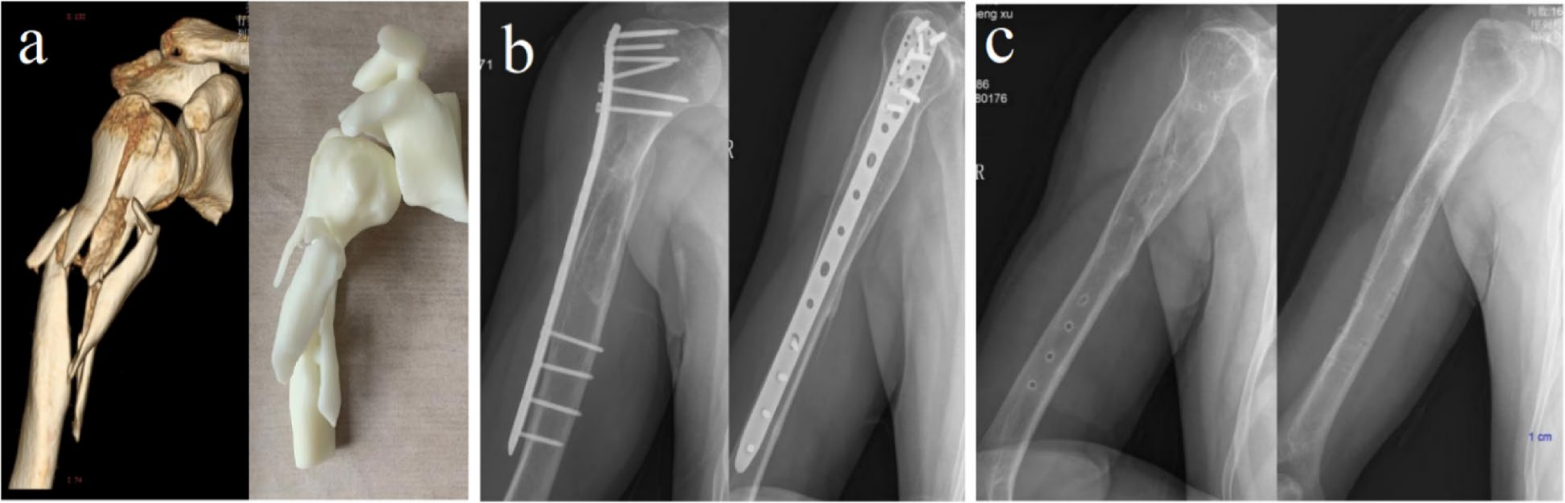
Case 2: a 41-year-old male, the AO/OTA classification was 12C13. a Preoperative 3D CT image and 3D anatomical model of middle-proximal humeral fracture. b The X-ray radiograph of full-length humerus 12 months after MIPO. c The X-ray radiograph of full-length humerus after removal of implants
